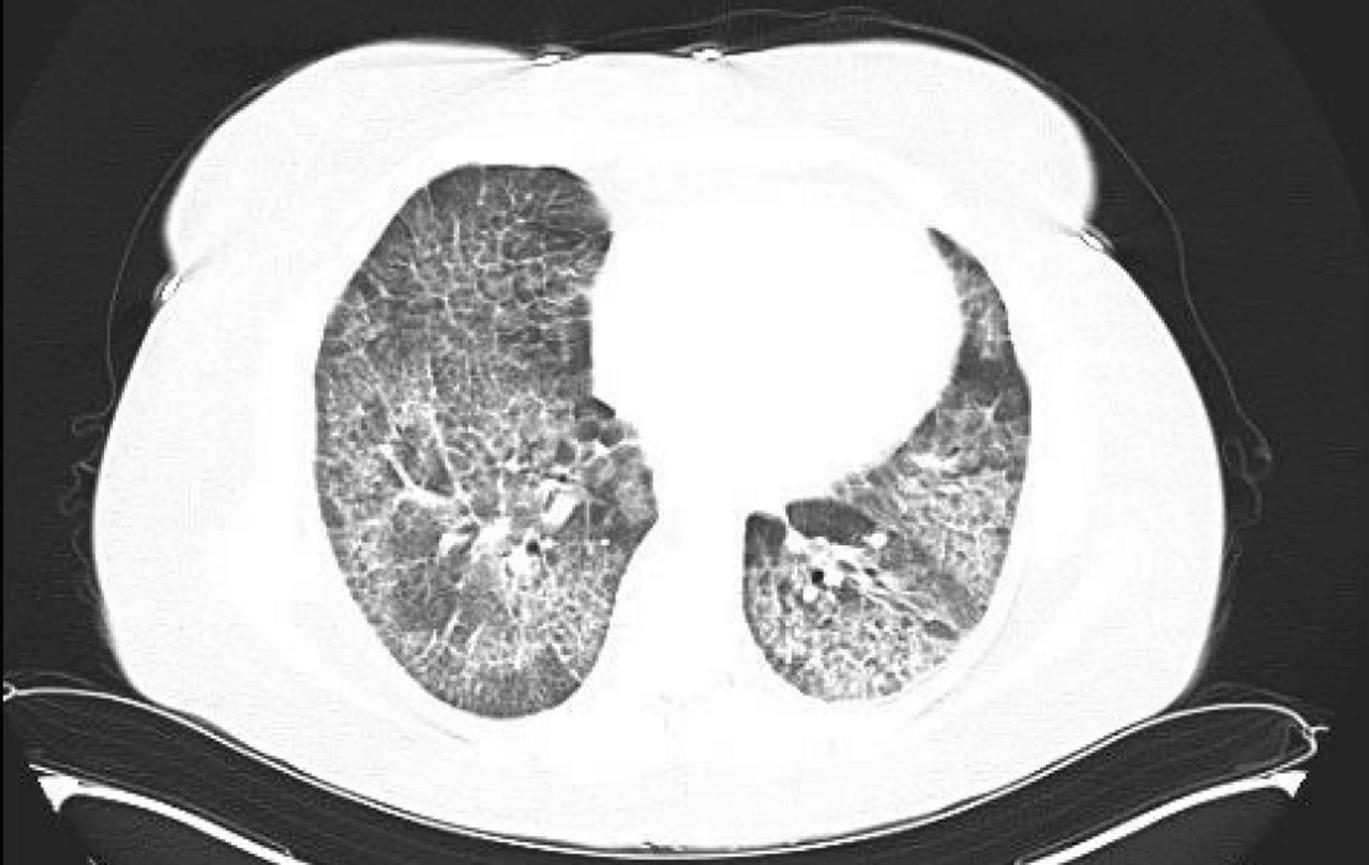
Computed tomography (CT) of chest showing a characteristic “crazy paving” appearance.
